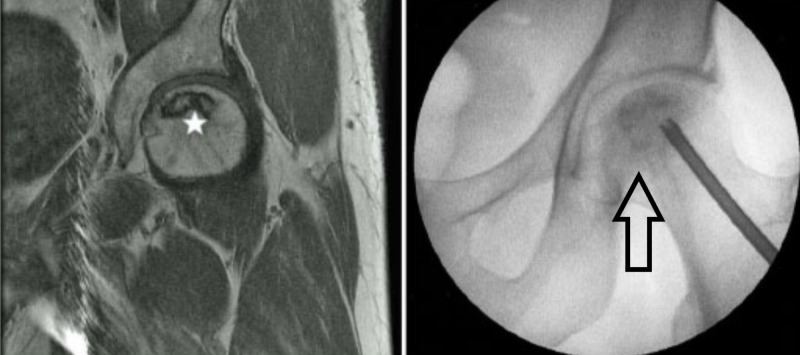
ONFH and decompression MRI with ONFH (left-sided image; star showing the osteonecrosis); X-ray left hip; arrow showing osteonecrosis and decompression. MRI: Magnetic resonance imaging; ONFH: Osteonecrosis of the femoral head
